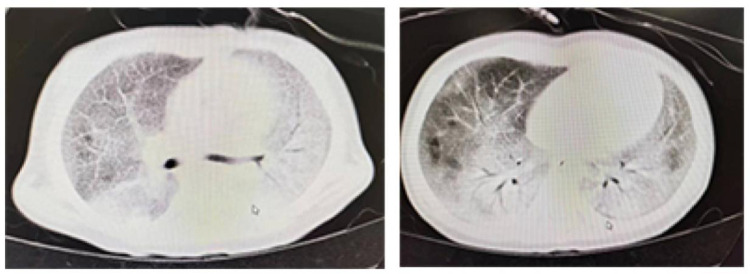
The preoperative lung CT scan of the patient showed a characteristic sign of pulmonary alveolar proteinosis, termed as “crazy paving pattern”, also known as “paving stone sign”.
